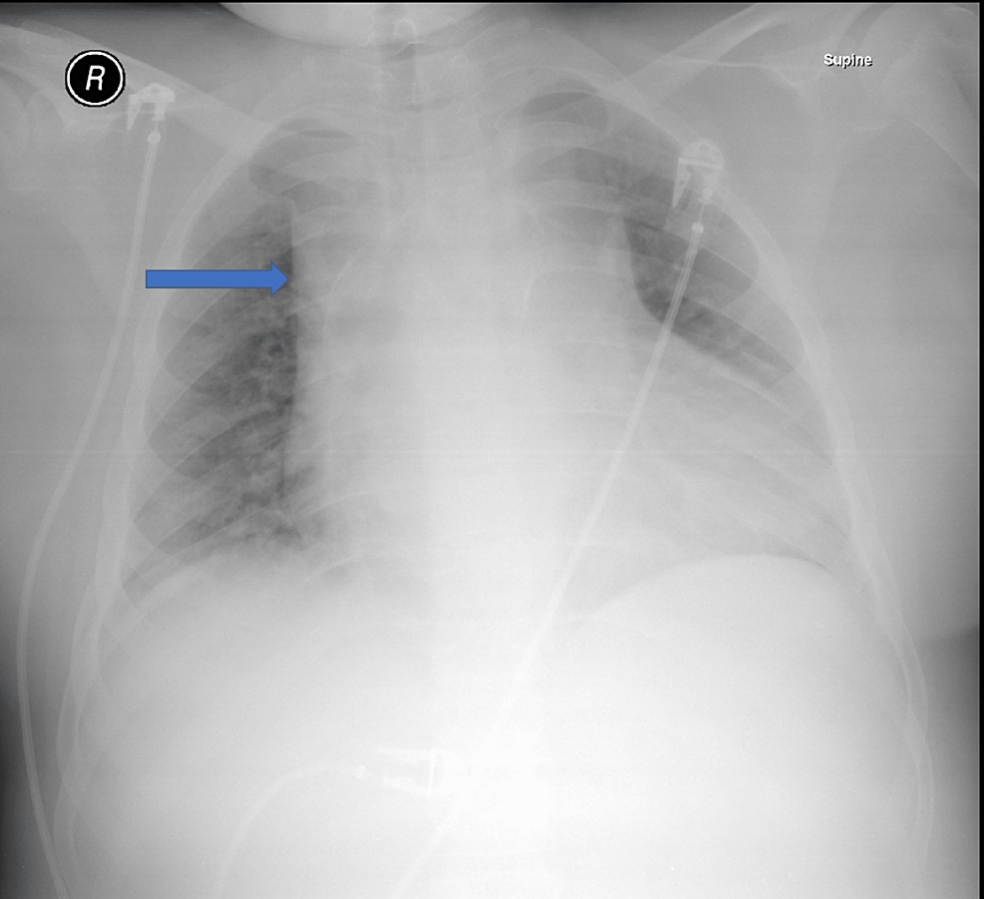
Portable X-ray of the chest
